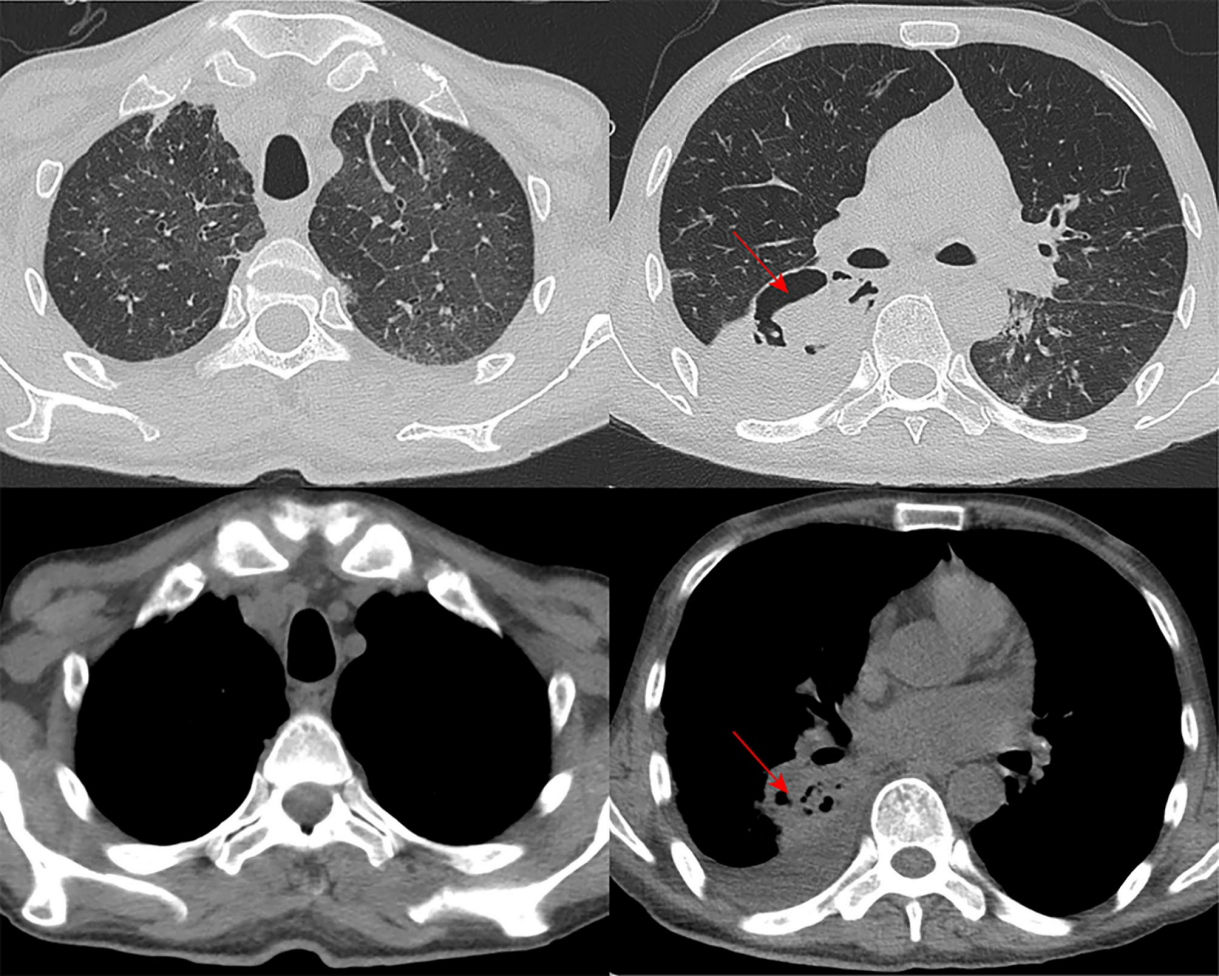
A follow-up chest CT after the patient was admitted to the hospital for treatment
